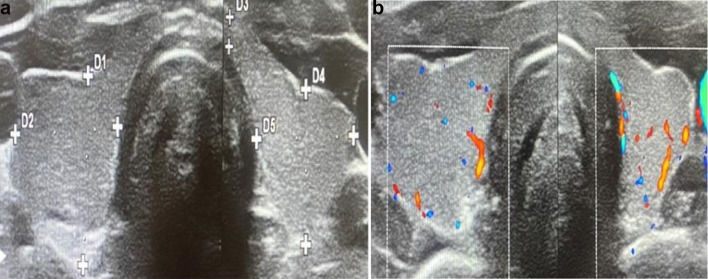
(a) Ultrasound examination of the thyroid gland with data on normal shape, size, localization, and ultrasound structure of the parenchyma. (b) Color Doppler with data on normal blood supply.
